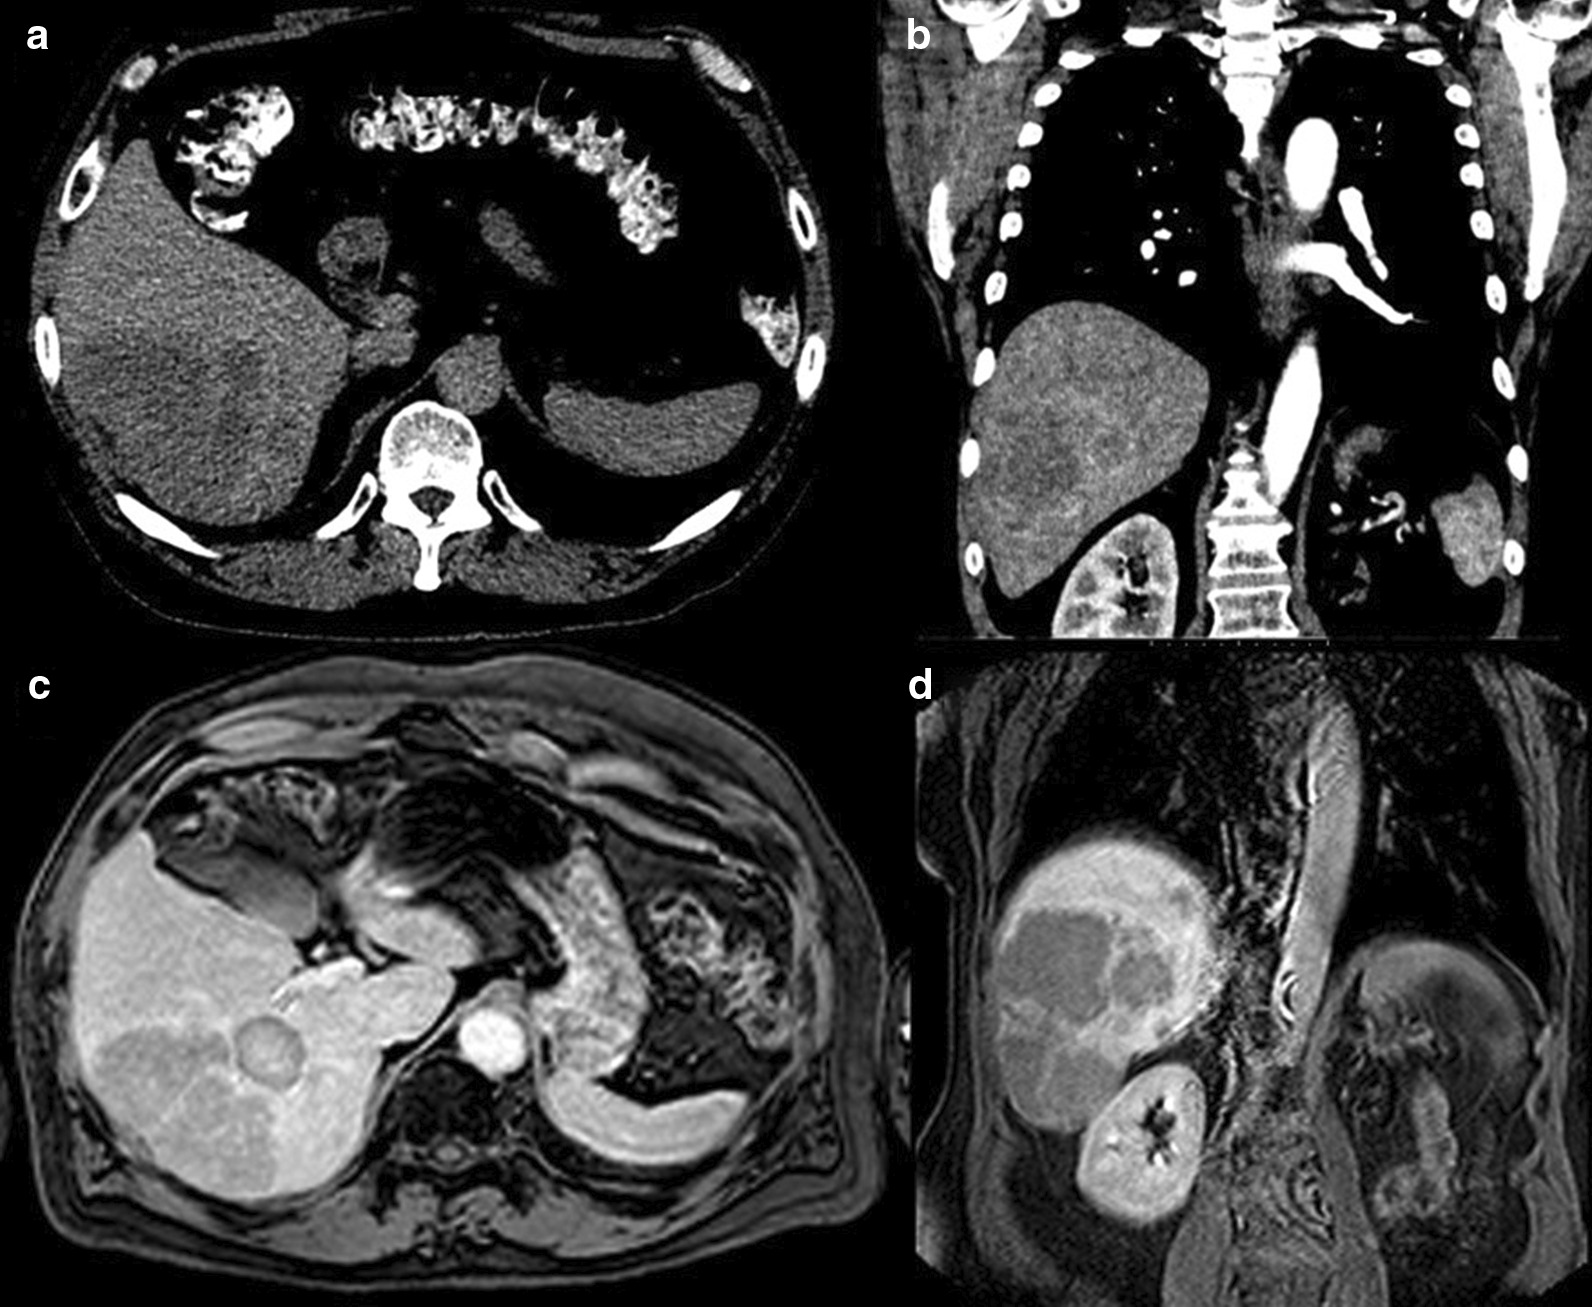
Metastatic adrenocortical carcinoma. a Six years after initial diagnosis, a surveillance non-contrast CT showed a lobulated, hypodense 9.5 × 8.7 cm hepatic segment 5/6 mass. b Contrast-enhanced CT showed peripheral enhancement with central necrosis, suspicious for metastases. c 3-min post-Eovist (Bayer, New Jersey, USA) T1-weighted fat-suppressed MRI showed multiple ring-enhancing right inferior hepatic lesions. d One month after attempted surgical resection, delayed post-Eovist T1-weighted fat-suppressed MRI showed enlarging right inferior hepatic masses
